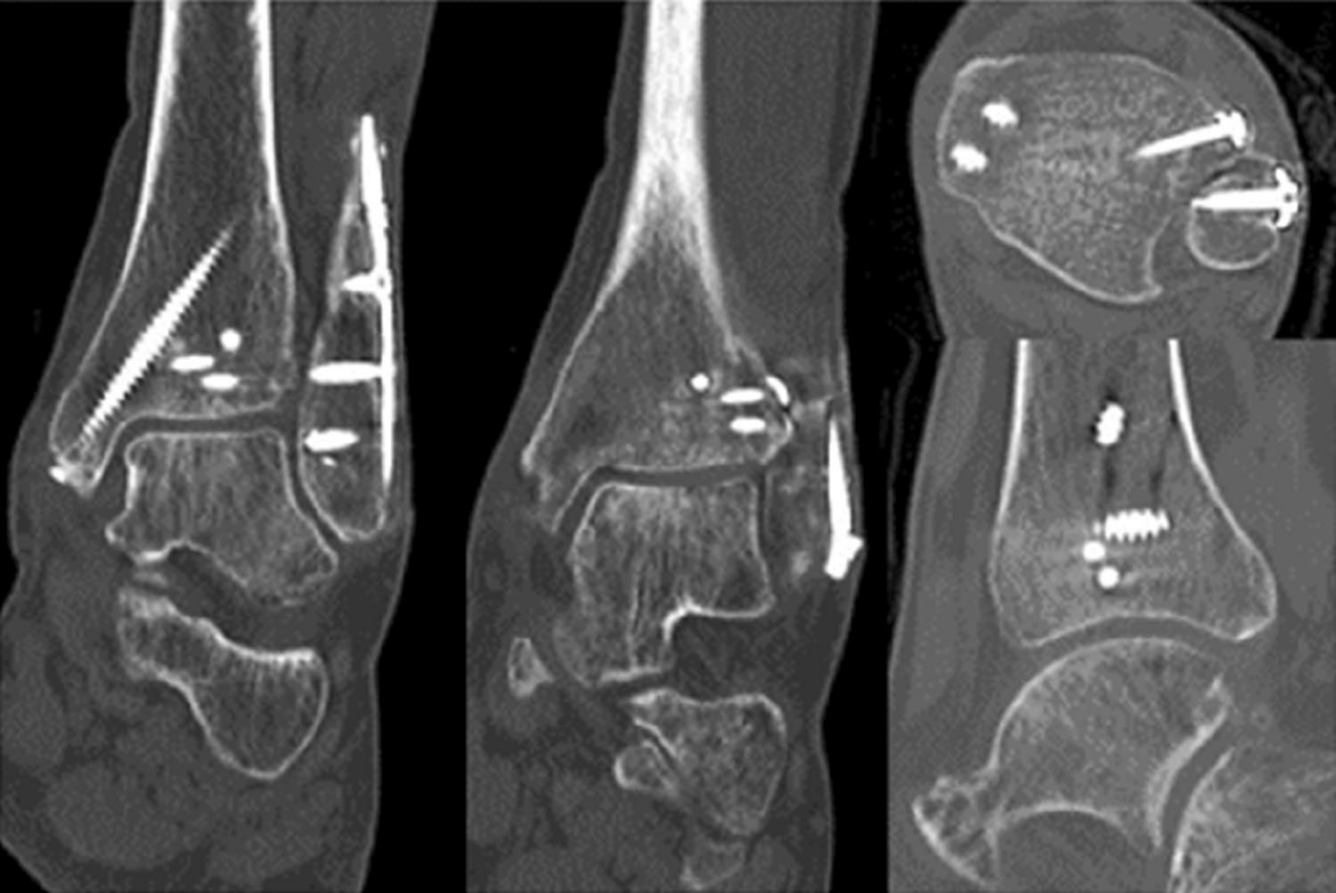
Postoperative CT imaging showing anatomic reduction with restoration of the incisura, the lateral plafond and ankle mortise (same patient in Figs. 1,2, 3)
